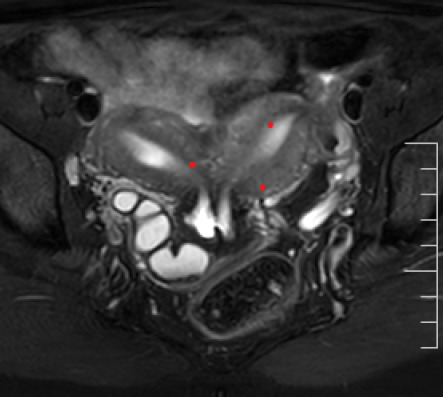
MRI showed uterine didelphys with the duplicated cervix and small communication exists between the two cervix. A cystic tubular structure was noted on the right side.
